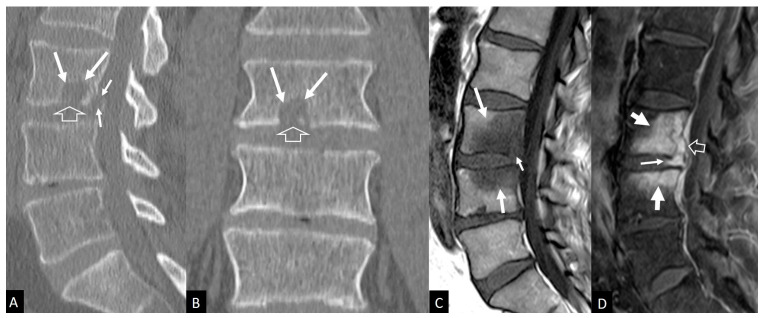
Staphylococcus aureus* spondylodiscitis in a 53-year-old male, with a history of microdiscectomy at the L3-L4 level, 6w prior to imaging. Sagittal (A) and coronal (B) CT reformatted images showing osteolysis in the lower L3 vertebral body (arrows) with cortical disruption of the lower epiphyseal plate (open arrows). An additional osteolysis with cortical disruption is shown posteriorly (short arrows). (C) Sagittal T1-w MR image showing bone marrow edema in the vertebral bodies L3 and L4 (arrows) and cortical disruption of the posterior epiphyseal plate (short arrow). (D) Fat suppressed contrast-enhanced sagittal MR image showing enhancement of the bone marrow (thick arrows) and the posterior disc (thin arrow). Intravertebral abscess formation is shown posteriorly (open arrow).
